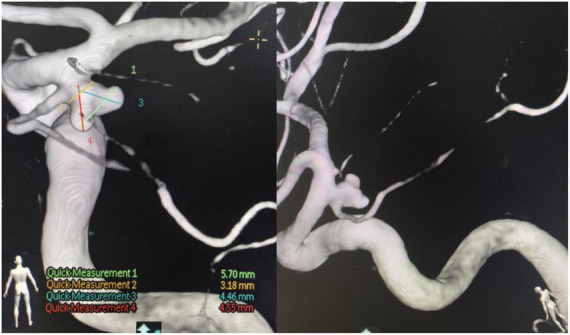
Postoperative non-contrast cranial computed tomography (CT) and CT angiography (CTA).
